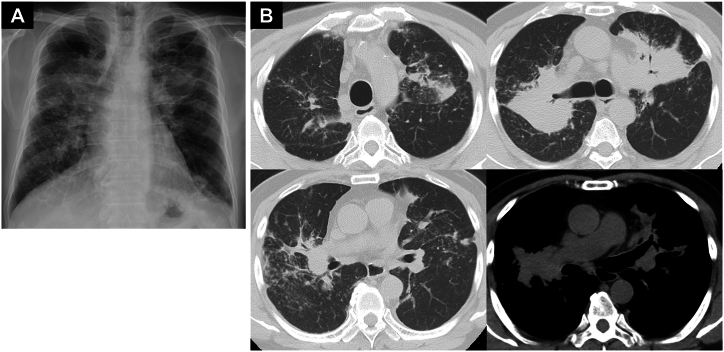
(A) Chest X-ray demonstrated tumor-like lesions in both lungs. (B) Chest computed tomography scan at the first examination demonstrated mediastinal and hilar lymph node swelling, bilateral tumor-like lesions, and granular shadows.
